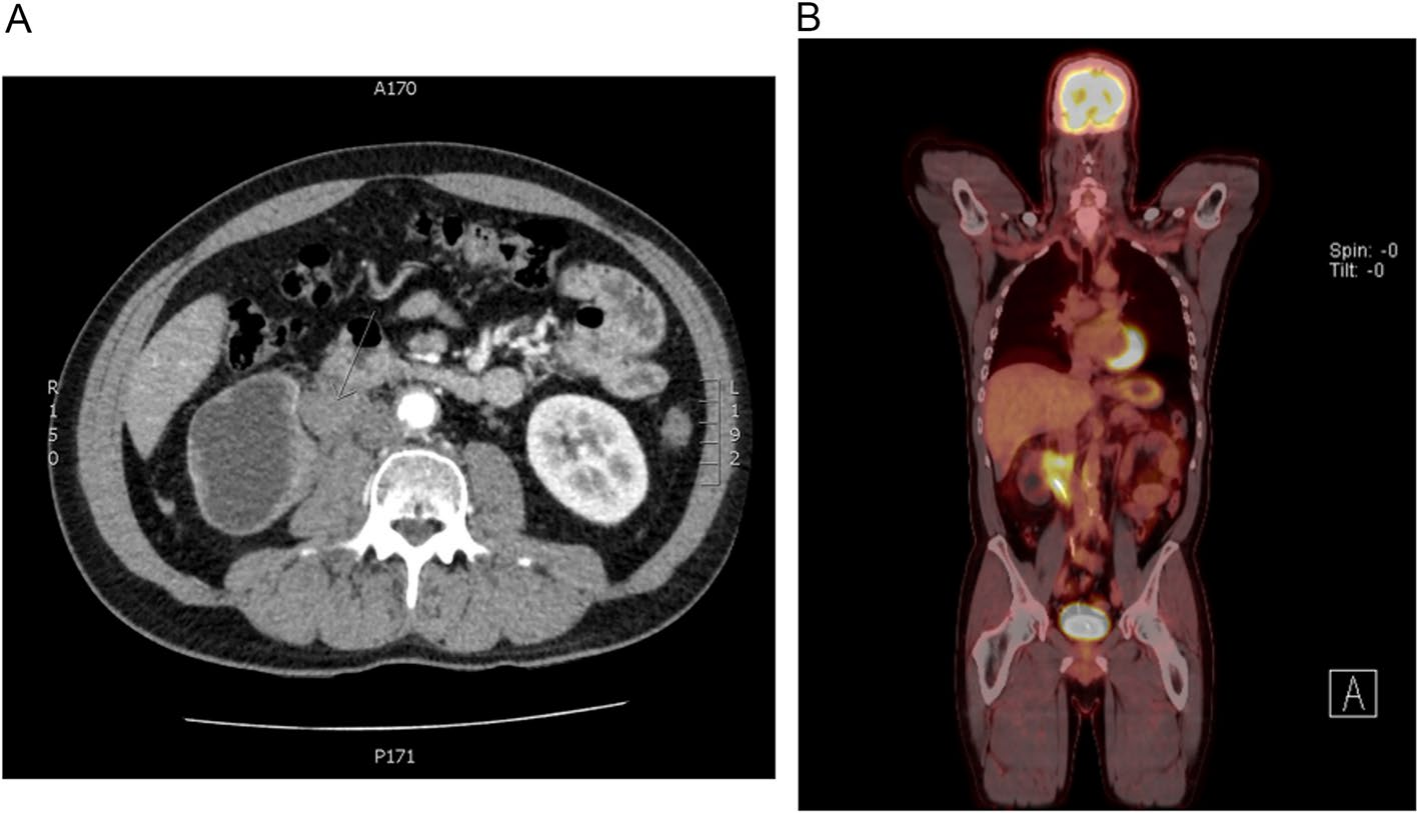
A CT imaging demonstrating the renal pelvic mass lesion, associated poor drainage and atrophy of the ipsilateral kidney. B PET imaging demonstrating intense FDG avidity of lesion in question
